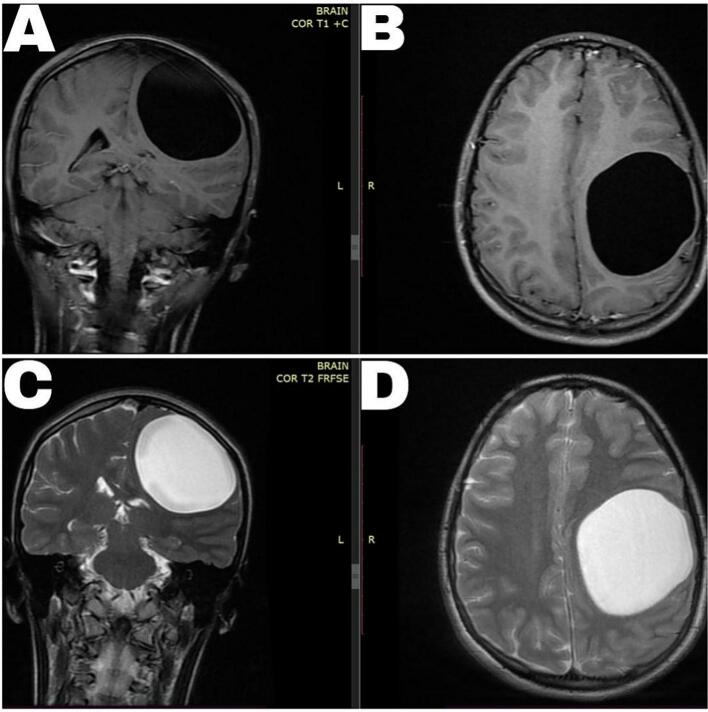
Pre-operative brain MRI with contrast. (A) T1 Coronal, (B) T1 Axial, (C) T2 Coronal and (D) T2 Axial. Reveal a well-defined, thin-walled cystic mass located in the left frontal, temporal, and parietal lobes. The mass is hypointense compared to the cerebral cortex on T1-weighted sequences and hyperintense on T2-weighted sequences.
